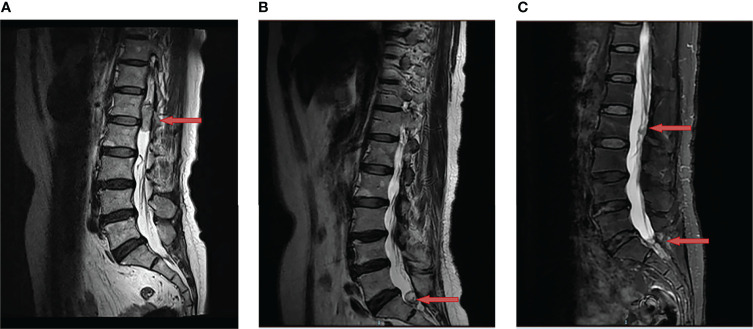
Changes of hemangioblastoma of the magnetic resonance imaging (MRI). (A) A space-occupying lesion at L1-L2 was detected (red arrow) in May 2016; (B) A recurrent site at S1 was observed (red arrow) after the first surgery in July 2019; (C) Two recurrent sites at L2 and S1-S2 were discovered (red arrow) after the second surgery in June 2020.
